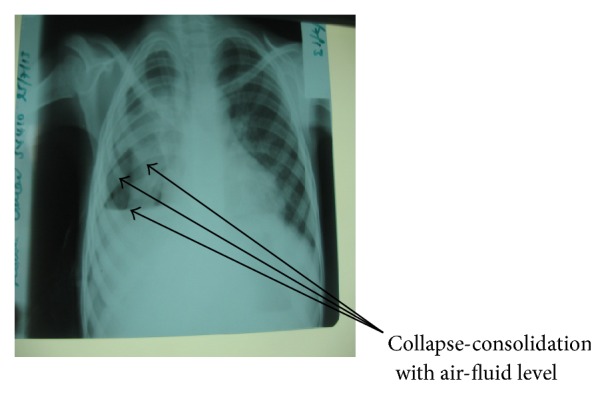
A chest X-ray after two-week course of antibiotics.
